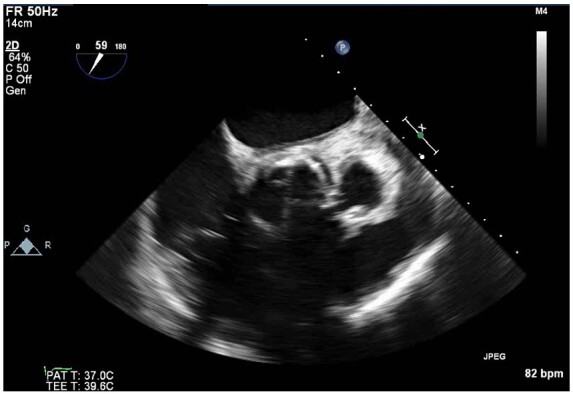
Transoesophageal echocardiogram mid-oesophageal short-axis aortic valve view showing peri-annular aortic root abscess.
